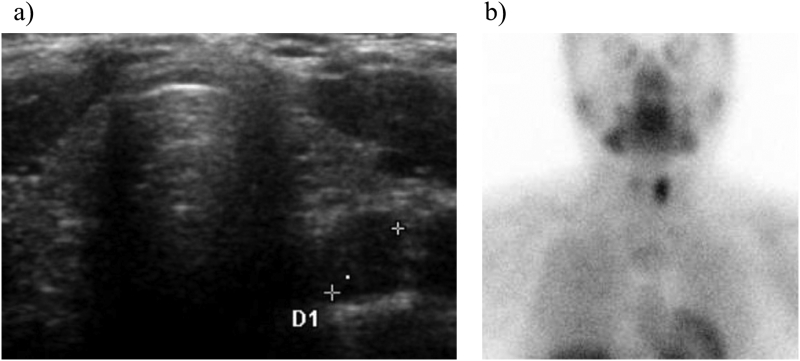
a) In case 1, ultrasonography revealed a 3.53 × 1.65 × 1.28 cm solid mass localized in the right inferior thyroid gland. b): ^99m^Tc-MIBI scintigraphy identified a hyperfunctioning right inferior parathyroid gland in case 1.
